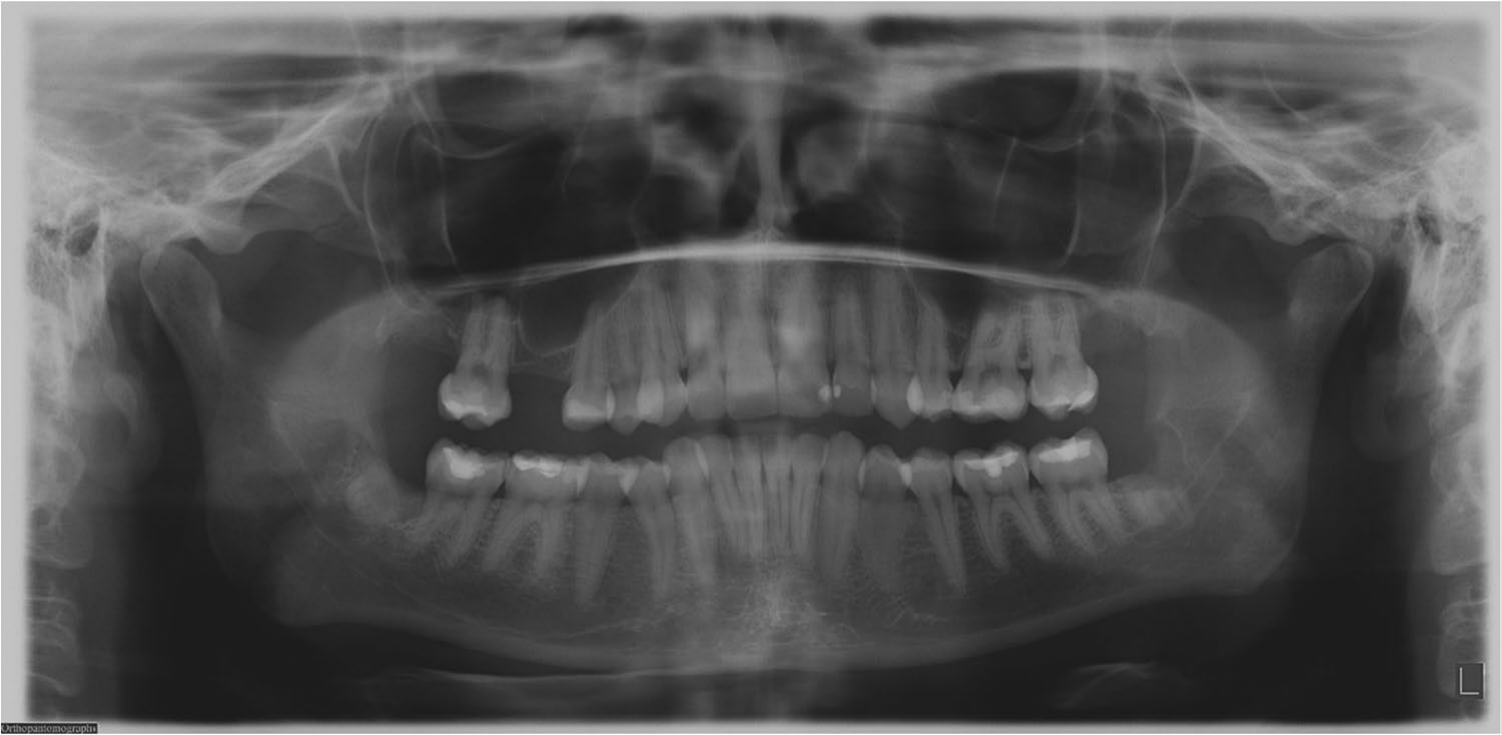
Panoramic radiograph of the same patient performed 6 months after coronectomy of the right mandibular third molar and coronectomy of the left mandibular third molar and removal of the left maxillary third molar
